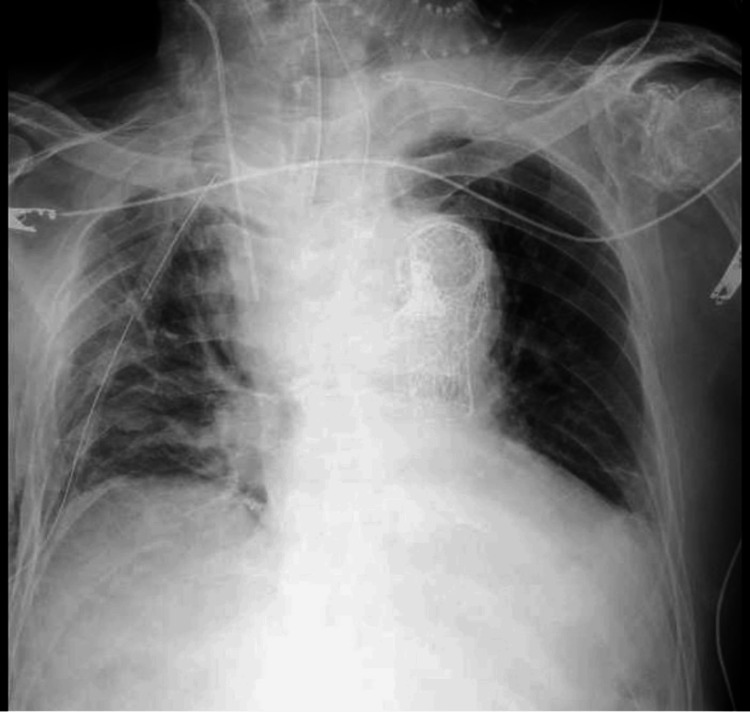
Portable chest X-ray Portable chest X-ray showing significant mediastinal widening in a patient with aortoesophageal fistula (AEF), suggesting possible aortic pathology and associated complications
